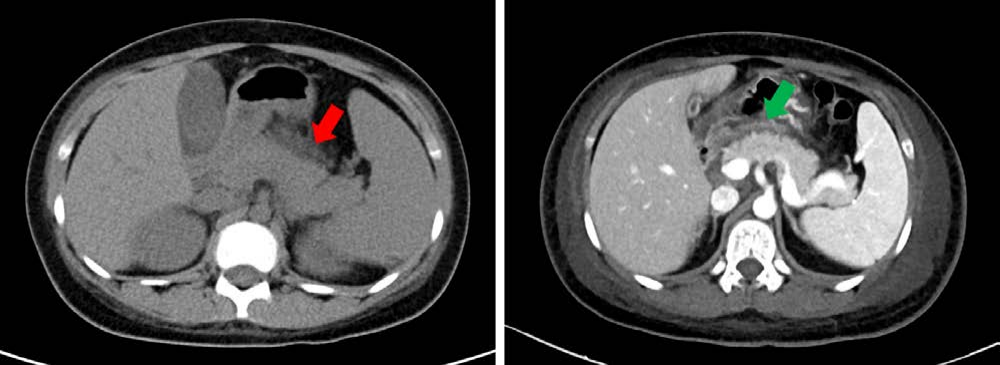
CT scan of the abdomen showed acute pancreatitis and hydrops abdominis, as shown by the red and green arrows.
